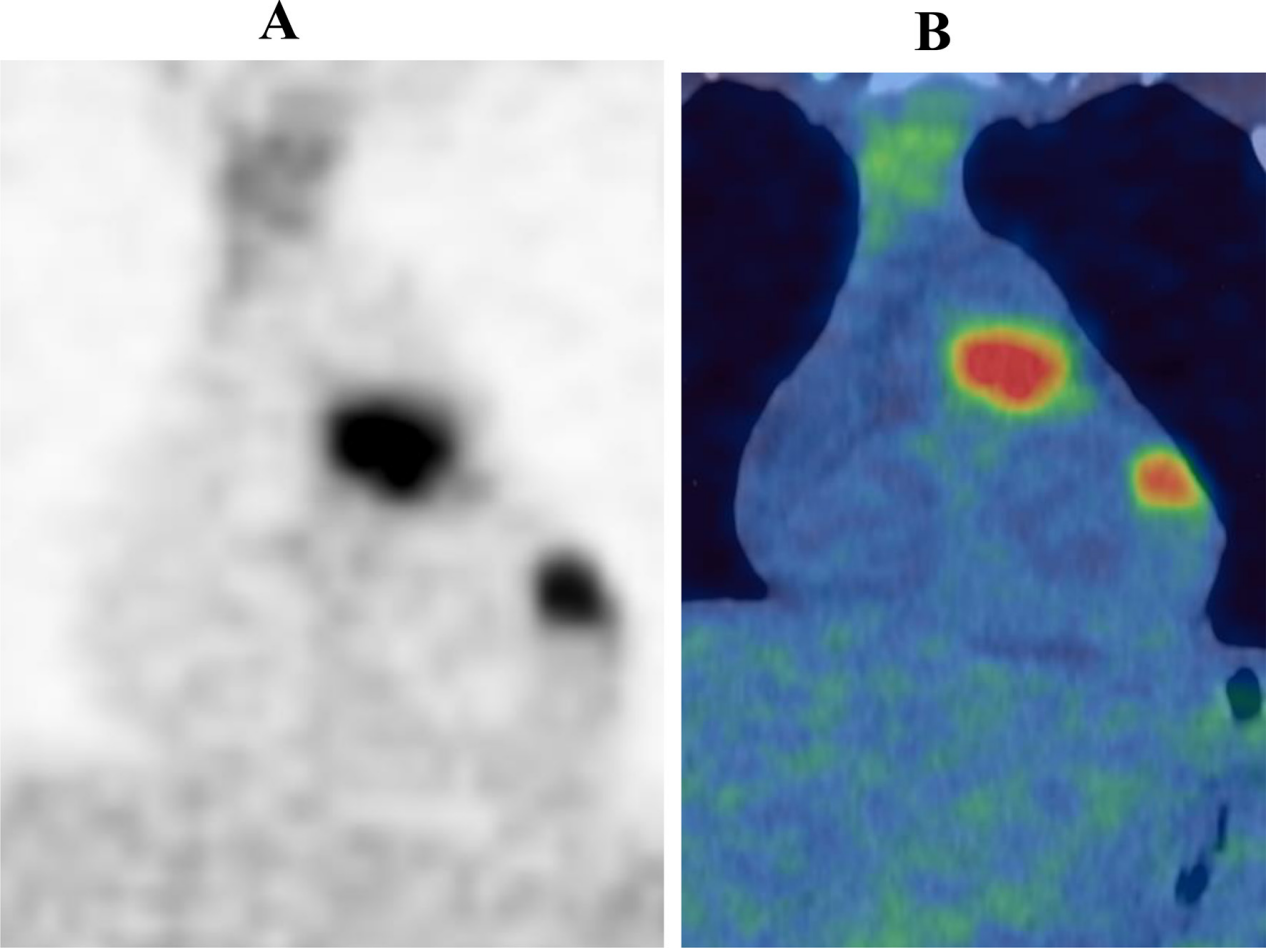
Coronal ^18^F-FDG PET only (A) and PET-CT fused (B) images demonstrating moderate uptake in right ventricular outflow tract lesion and left ventricular lesion. ^18^F-FDG PET, 18-fludeoxyglucose positron emission tomography.
